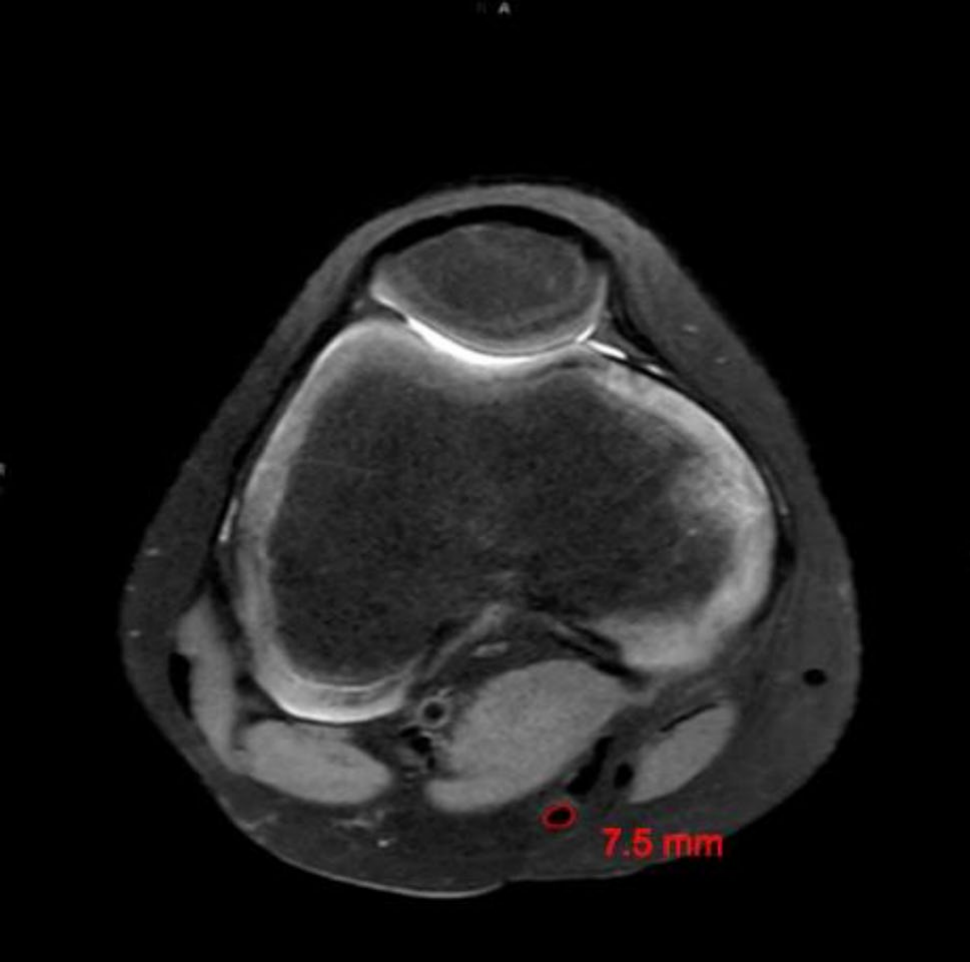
Cross-sectional area (CSA) of the semitendinosus tendon (ST) on an axial MRI (T2 sequence) of a patient with a weight of 35 kg showing a CSA of the semitendinosus tendon of 7.5 mm^2^
